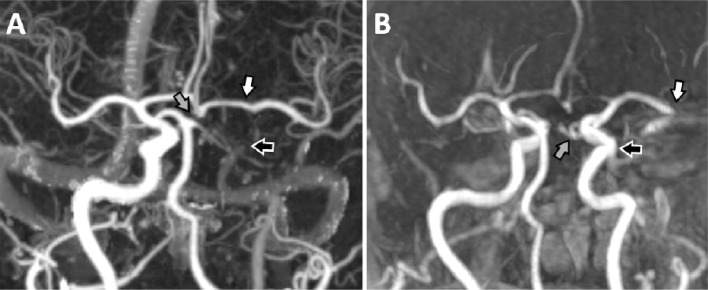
Case 1 - CTA (A) of case 1 performed 3 hours after symptom onset. An occlusion of the left intracranial ICA (black arrow) with preserved filling of the left MCA (white arrow) was visible. Note the missing depiction of the left PCA (grey arrow). MRA (B) performed right before thrombectomy revealed a complete recanalisation of the left ICA (black arrow) and partial filling of the left fPCA (grey arrow). Migration of the initial thrombus to the major central M2-branch of the left MCA (white arrow). CTA, CT-angiography; fPCA, fetal posterior cerebral artery; ICA, internal carotid artery; MCA, middle cerebral artery; MRA, MR angiography.
